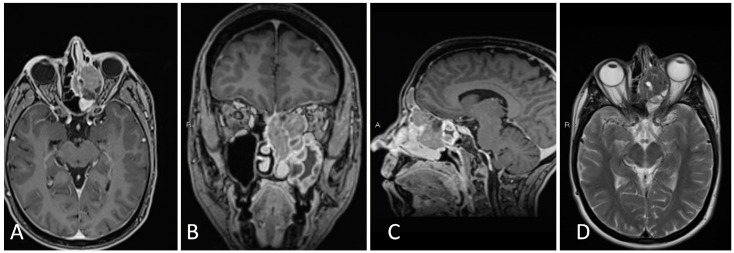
Preoperative imaging of a 44-year-old female with biopsy-proven squamous cell carcinoma of the left ethmoid, including invasion into the skull base and left lamina paprycea. (A) Axial T1 post-contrast MRI. (B) Coronal T1 post-contrast MRI. (C) Sagittal T1 post-contrast MRI. (D) Axial T2-weighted MRI.
